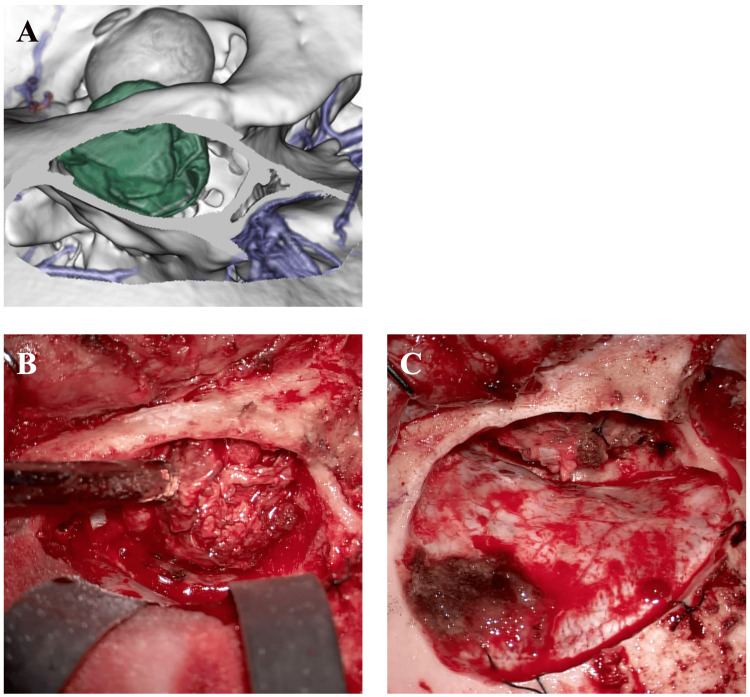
Intraoperative view (A) 3D reconstruction from computed tomography (CT) scans; (B) pre-resection view; (C) post-resection view. A right frontotemporal craniotomy was performed to access the superolateral orbit, including resection of the orbital roof.
